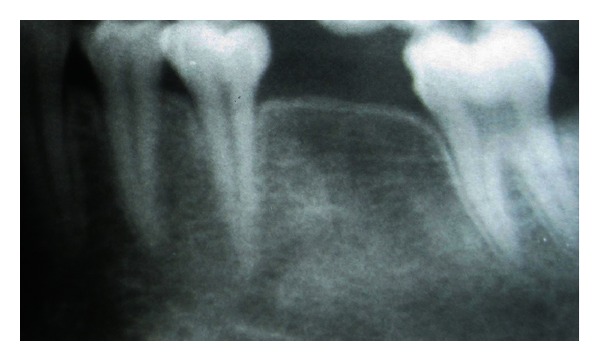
Preoperative radiograph of tooth, number 36, note the good height of the alveolar bone.
